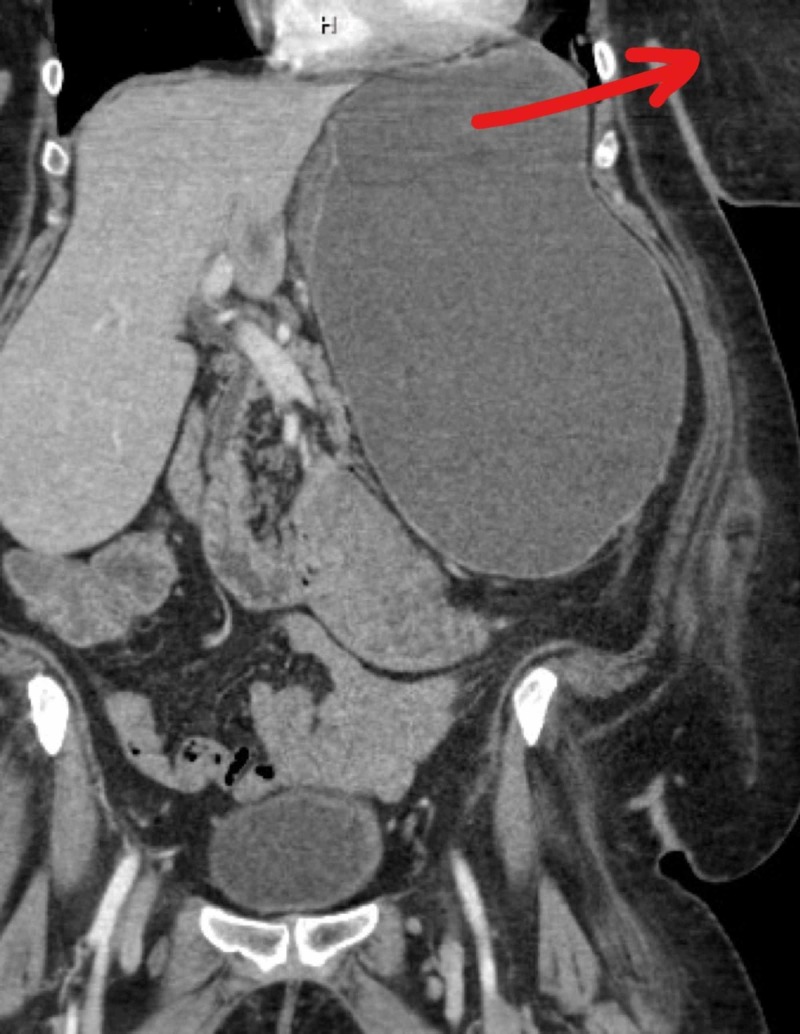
CT abdomen and pelvis CT scan abdomen, coronal view showing large parastomal hernia (Red arrow indicating location of the sac).
